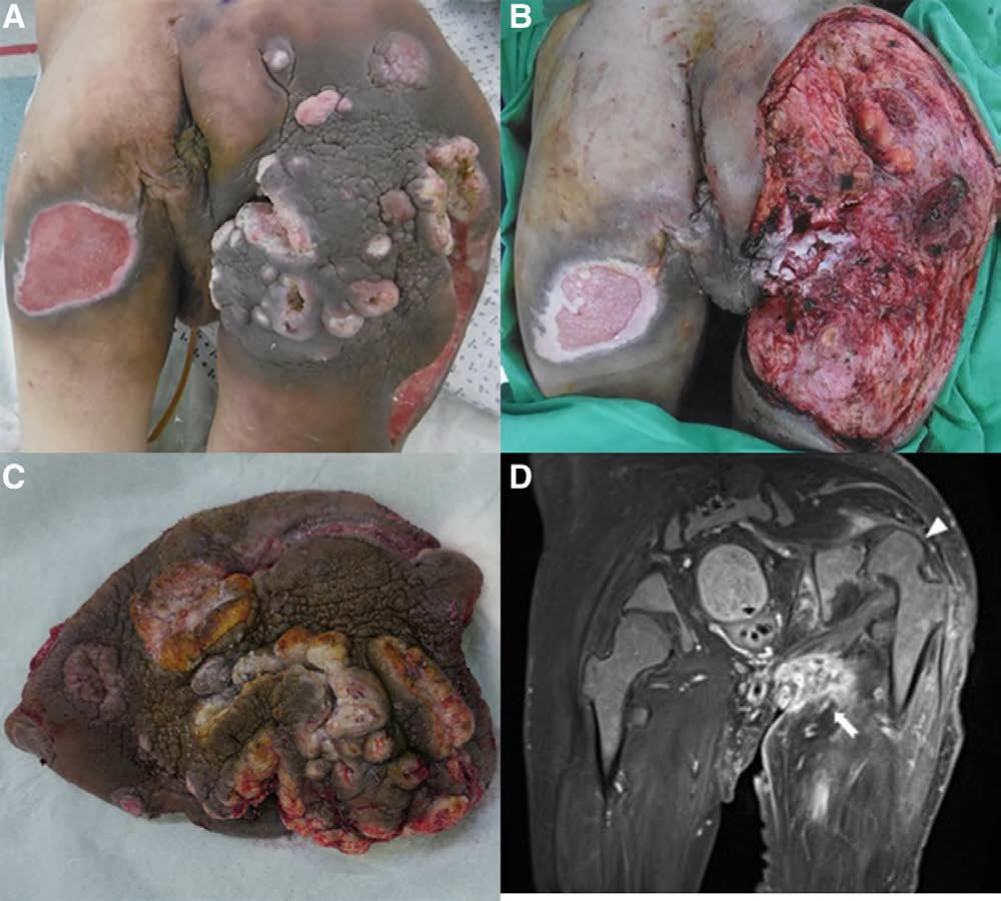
(A) Clinical picture post-serial debridement and 2-week antibiotic treatment. (B, C) Wide excision of the mass that was later confirmed as a squamous cell carcinoma. The dissection depth included a part of the gluteal muscle, and the ischium was exposed. (D) Postoperative gadolinium-enhanced T1-weighted magnetic resonance image revealed residual infiltrative ulcerative tumor involving the internal obturator and gluteus maximus muscles. (Arrow) Cephalic dislocation of the right hip joint (arrowhead).
